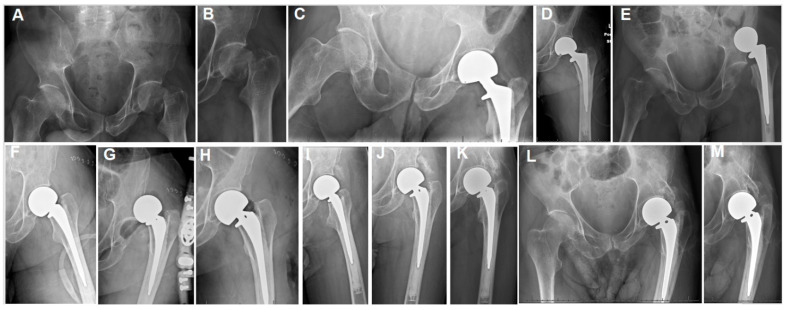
(A,B) The plain hip radiographs of a 55-year-old man who presented with a displaced left femoral neck fracture. (C,D) Postoperative plain hip radiographs taken immediately after cemented bipolar hemiarthroplasty. (E) One-week postoperative plain pelvic radiographs showing dislocated HA and stem loosening. (F) Plain hip AP radiographs after open revision. (G) A plain hip radiograph taken one day postoperatively showing re-dislocation. (H) Closed reduction was performed. (I) A plain hip radiograph taken at 4 weeks after the initial HA surgery, showing early HO lateral to the acetabular margin, along with stable HA components. (J) Eight-week and (K) 40-month postoperative plain radiographs showing progressive heterotopic ossification (HO). (L,M) Seventy-two-month postoperative radiographs showing class 3 acetabular and proximal femoral HO.
